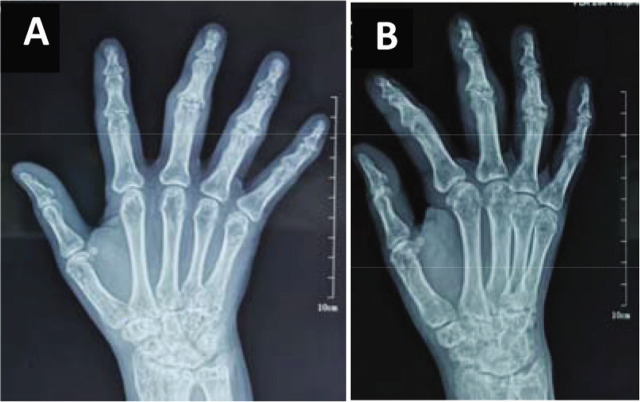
Radiography of the right-hand suggested osteoporotic looseness, joint space narrowing, and the second and third proximal interphalangeal joints bone destruction. A, positive position; B, lateral position
